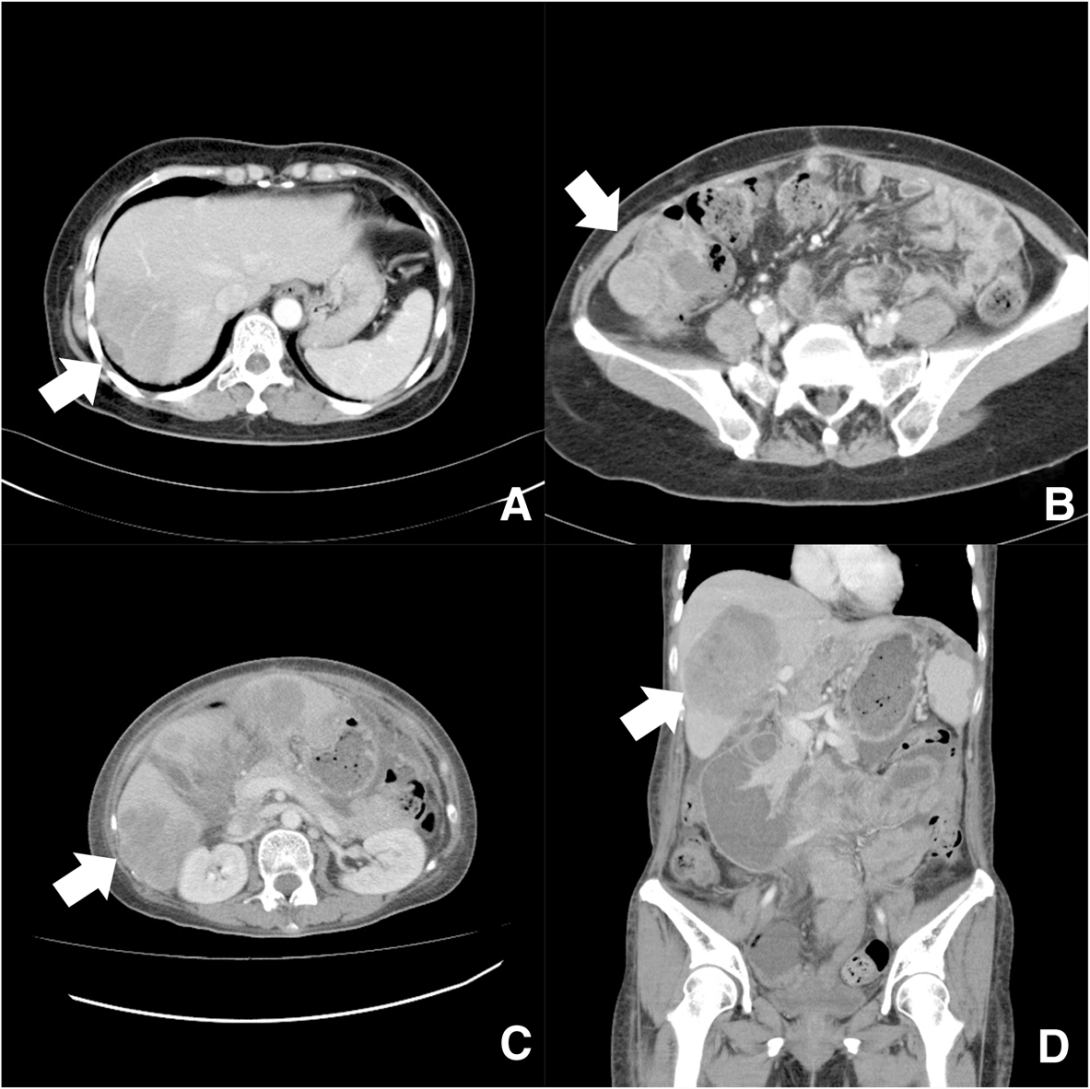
Computed tomography (a) axial view showing a 2-cm hypodense nodule at the right subphrenic region and wedge-shaped hypoperfusion over segment 7 of the liver suspicious tumor seeding and liver metastasis. b Coronal view showing a lobulated mixed solid and cystic lesion at the right paracolic gutter measuring 5 cm in size in favor of peritoneal seeding. c Axial and (d) coronal view on further abdominal CT follow-up showing tumor recurrence of the liver and increased tumor burden after 18 months of tertiary debulking surgery
